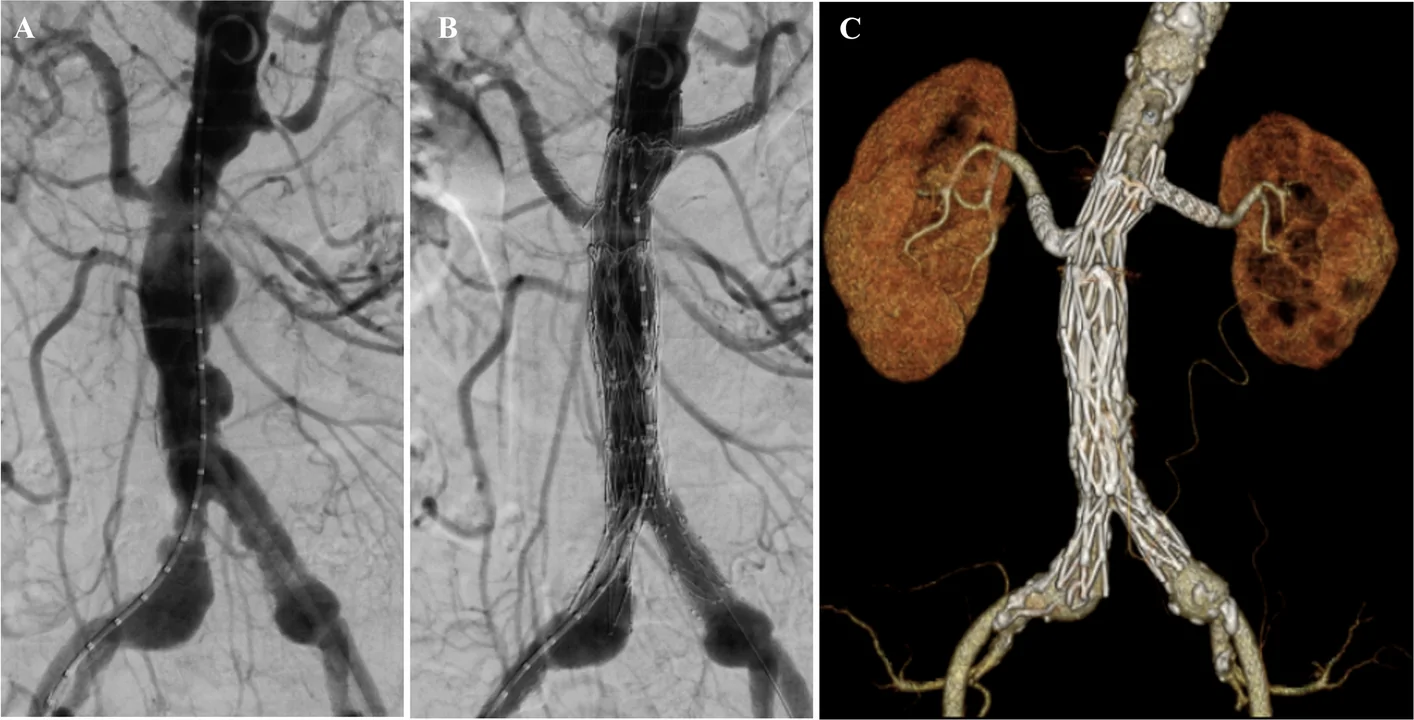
Intraoperative angiography and postoperative three-dimensional computed tomography angiography (CTA) (Case 1). A Initial intraoperative angiogram demonstrating the target pathology. B Completion angiogram confirming successful aneurysm exclusion and unimpeded flow through the target vessel, without evidence of endoleaks. C Postoperative three-dimensional CTA reconstruction demonstrating stable endograft conformation, a patent bridging stent, and complete exclusion of the aneurysm sac
